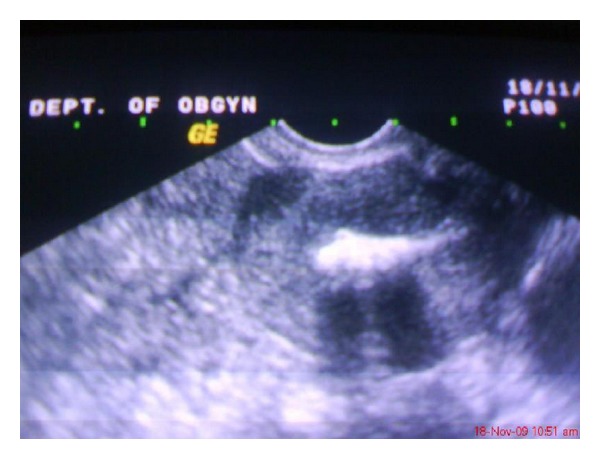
Transvaginal ultrasonography of the uterus revealed calcific areas.
